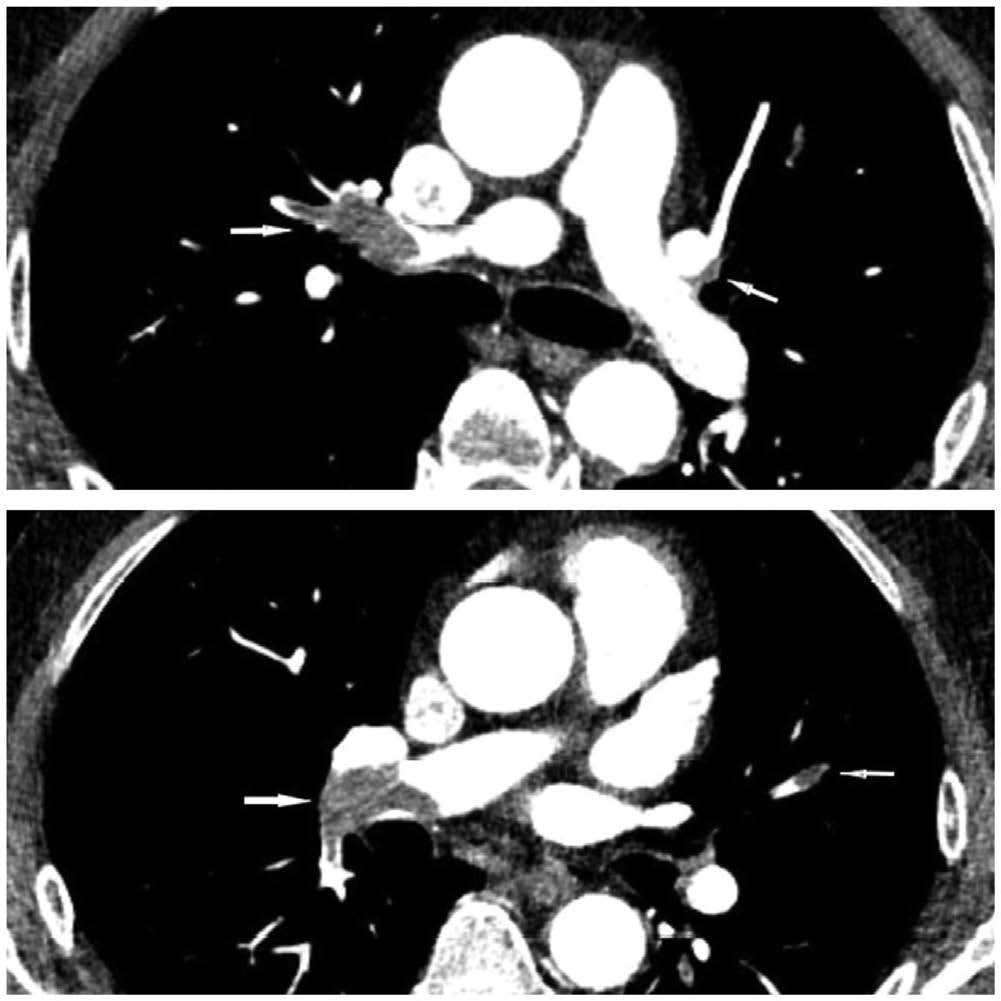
CTA of thoracic aorta showed bilateral multiple pulmonary embolism (white arrow). CTA = computed tomography angiography.
